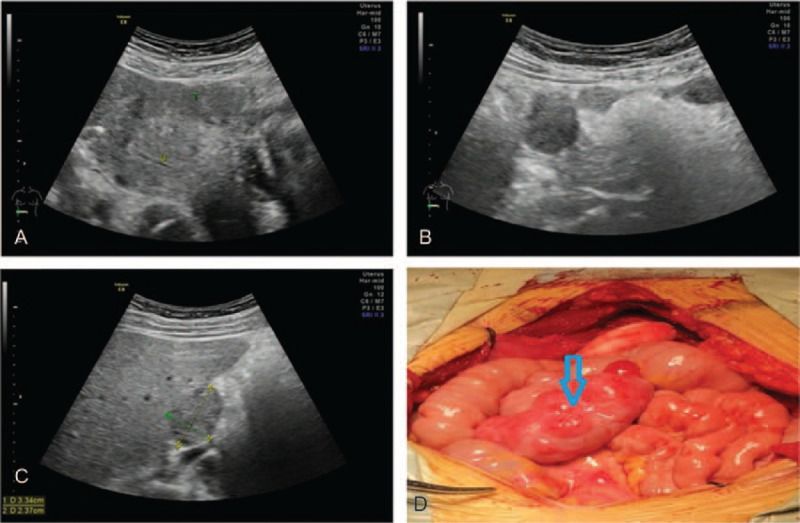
Ultrasound and intraoperative images of Case 2. A, B, Ultrasound showed multiple masses in the pelvic and abdomen. C, Ultrasound showed a hypoechoic nodule on lower edge of the right hepatic lobe. D, Multiple bead-like hard nodules on the surface of small intestine near to ileocecal junction.
